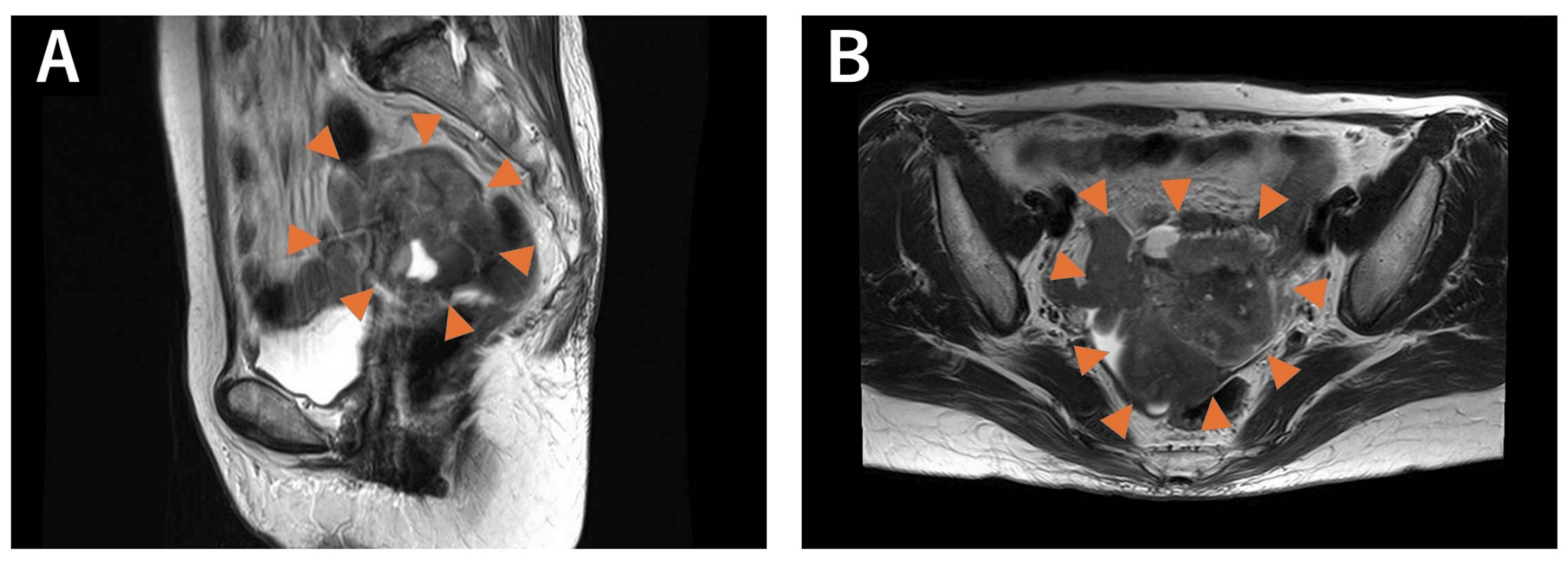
Pelvic contrast-enhanced MRI of case two Axial (A) and sagittal (B) T2WI revealed a mass in the left pelvis containing both solid and cystic components. The anterior part appeared solid, with mildly heterogeneous features and slightly high signal intensity on T2WI, whereas the posterior and medial parts appeared cystic, exhibiting low signal intensity on T2WI.
